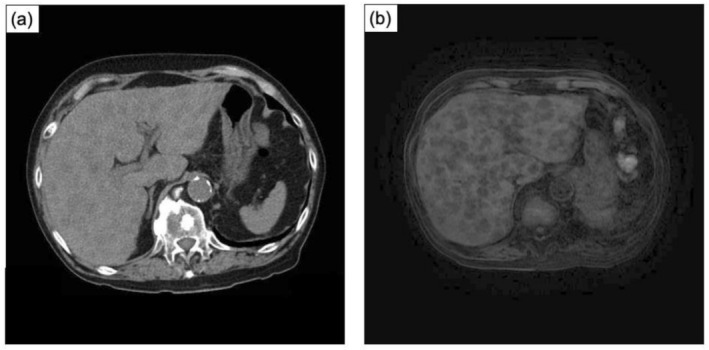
Plain computed tomography (a) and plain magnetic resonance image (b) scans showing multiple liver metastasis.
